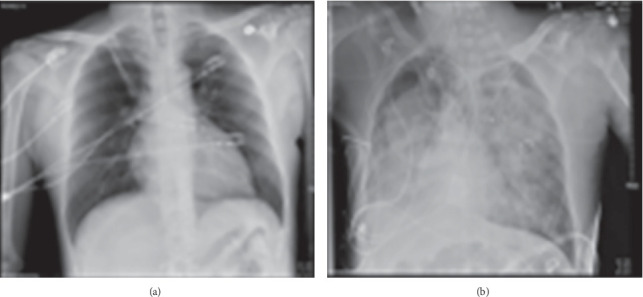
Chest X-rays demonstrating the rapid development of ARDS in Case 2. (a) Chest X-ray taken immediately after the patient underwent surgery demonstrating healthy lungs without evidence of infection. (b) On Postoperative Day 3, chest X-ray was consistent with ARDS.
